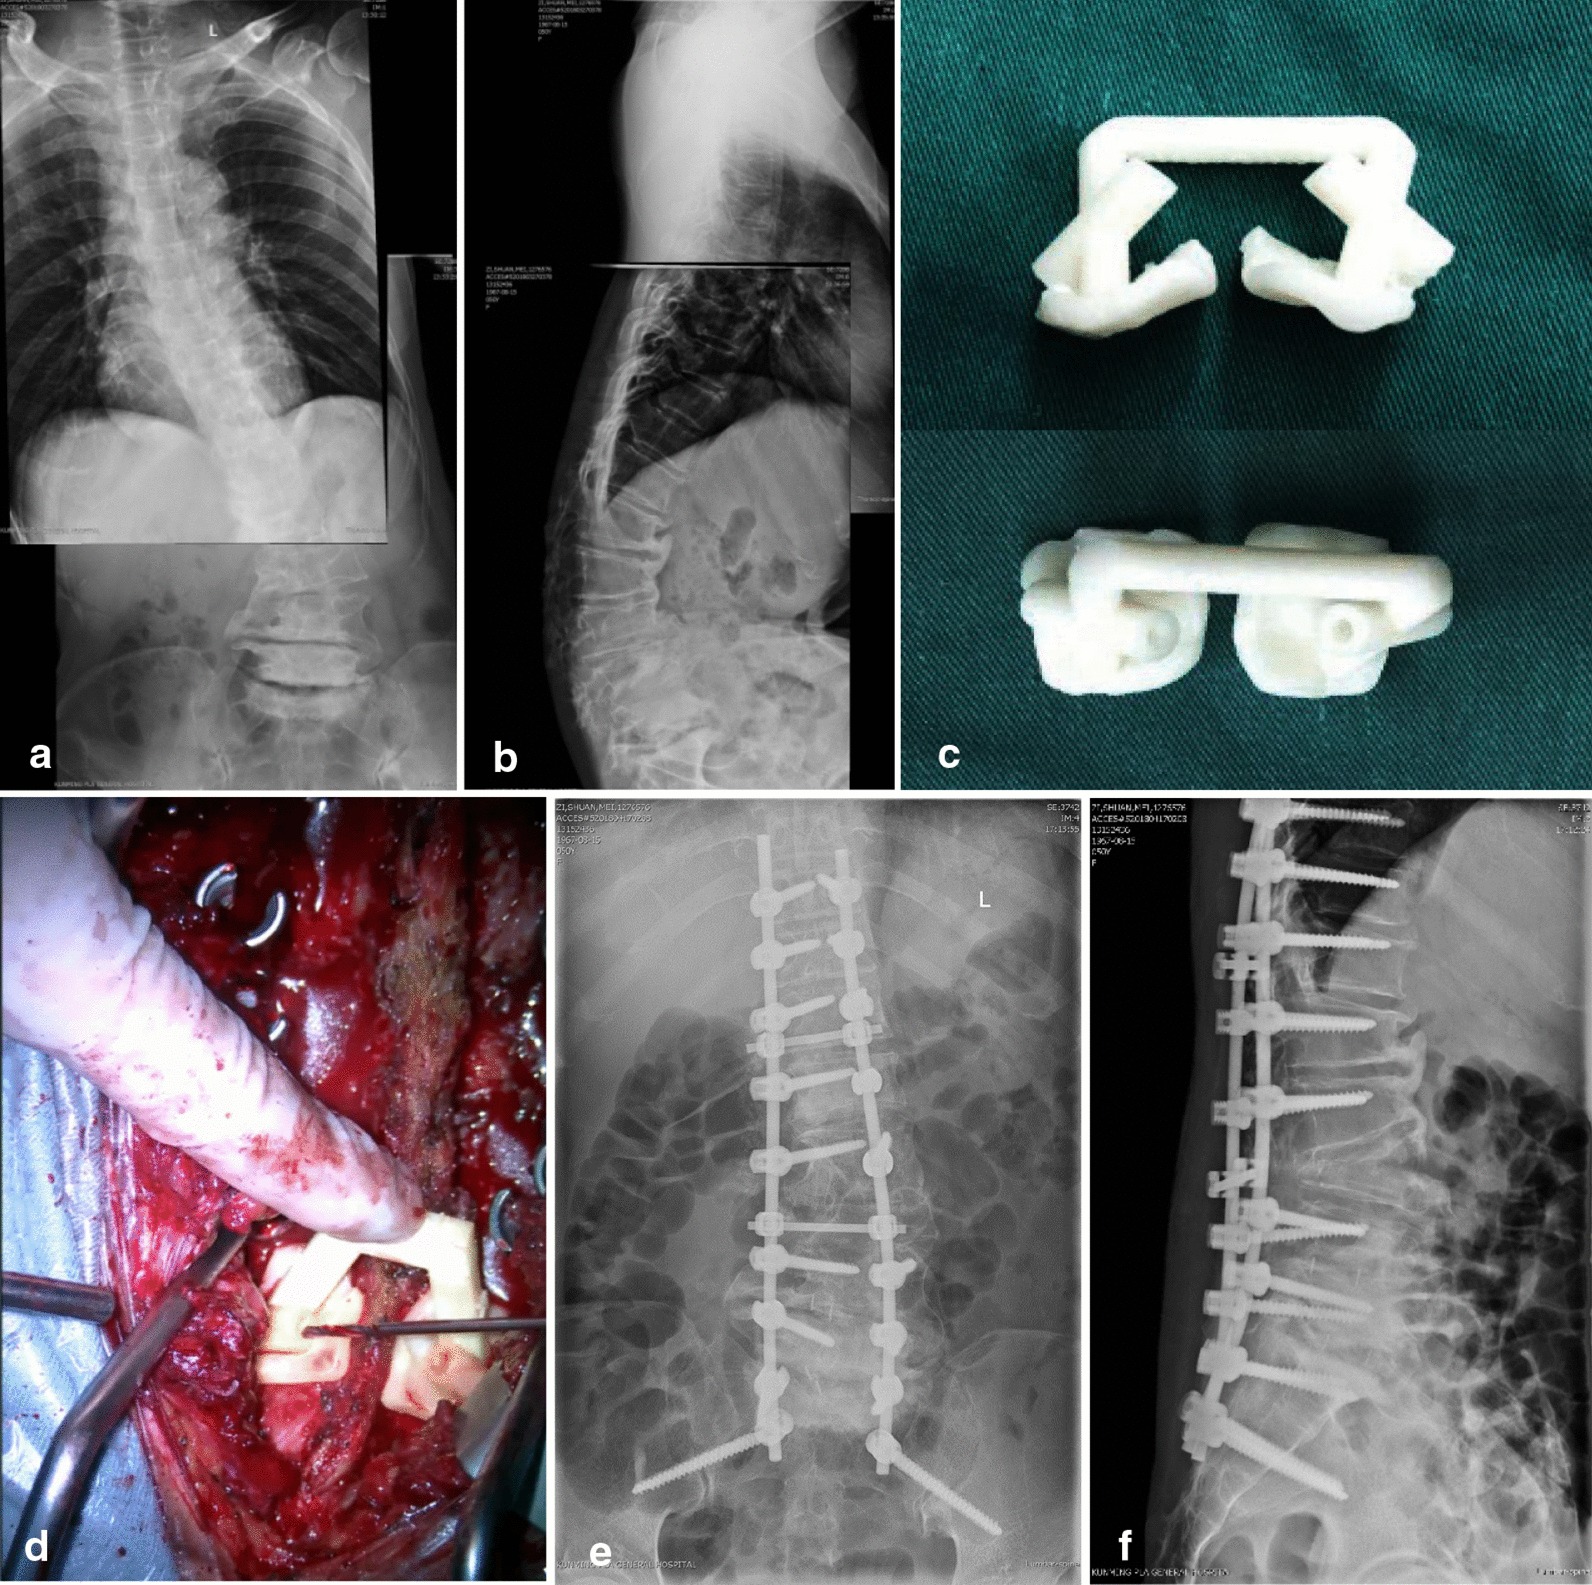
A 53-year-old woman with adult degenerative scoliosis. Anteroposterior-view radiograph (a) and lateral-view radiograph (b) before surgery. Low-temperature-plasma sterilization of the S2AI operation guide template before surgery (c). During the operation, the operation guide template was attached to the bone surface and secured for drilling, tapping, and screw placement along the axis of the screw trajectory (d). Postoperative follow-up anteroposterior (e) and lateral views (f) show that the deformity was well corrected, and the S2AI screw and other screws were in the desired places
